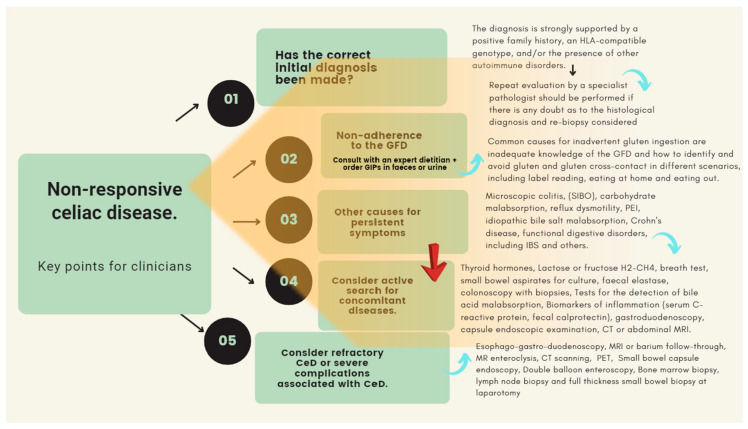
Approach to a patient with non-responsive CeD. Abbreviations: CT: computed tomography; MRI: magnetic resonance imaging; PET: positron emission tomography; PEI: pancreatic exocrine insufficiency.
